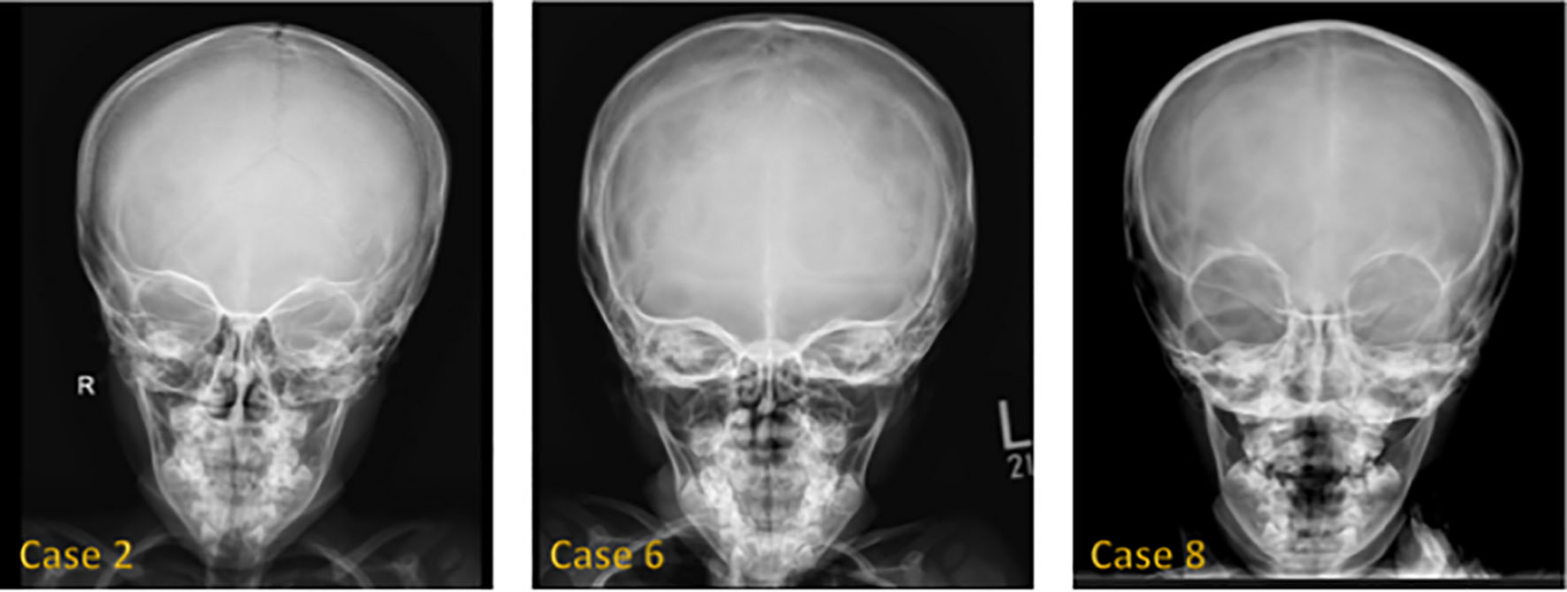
Frontal face radiograph showed a triangular configuration of the face in Cases 2, 6, and 8. Case 8 also showed maxillary hypoplasia, teeth malocclusion, and teeth crowding.
